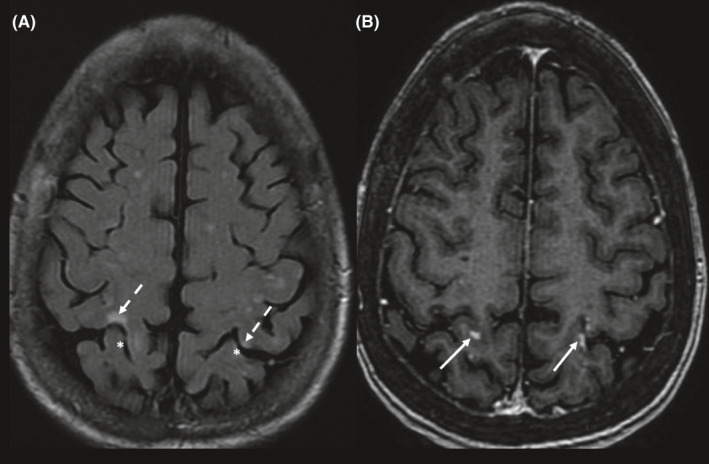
Brain MRI with gadolinium, showing cortical lesions of increased T2 signal (A) and enhancement (B, arrows). Note cortical lesion in A (dashed arrows) is none enhancing in B, while the enhancing cortical lesions in B (arrows) show almost no increased T2 signal on A (asterisks). These imaging features are inconsistent with brain metastases in which cortical T2 hyperintensity and enhancement are matching
